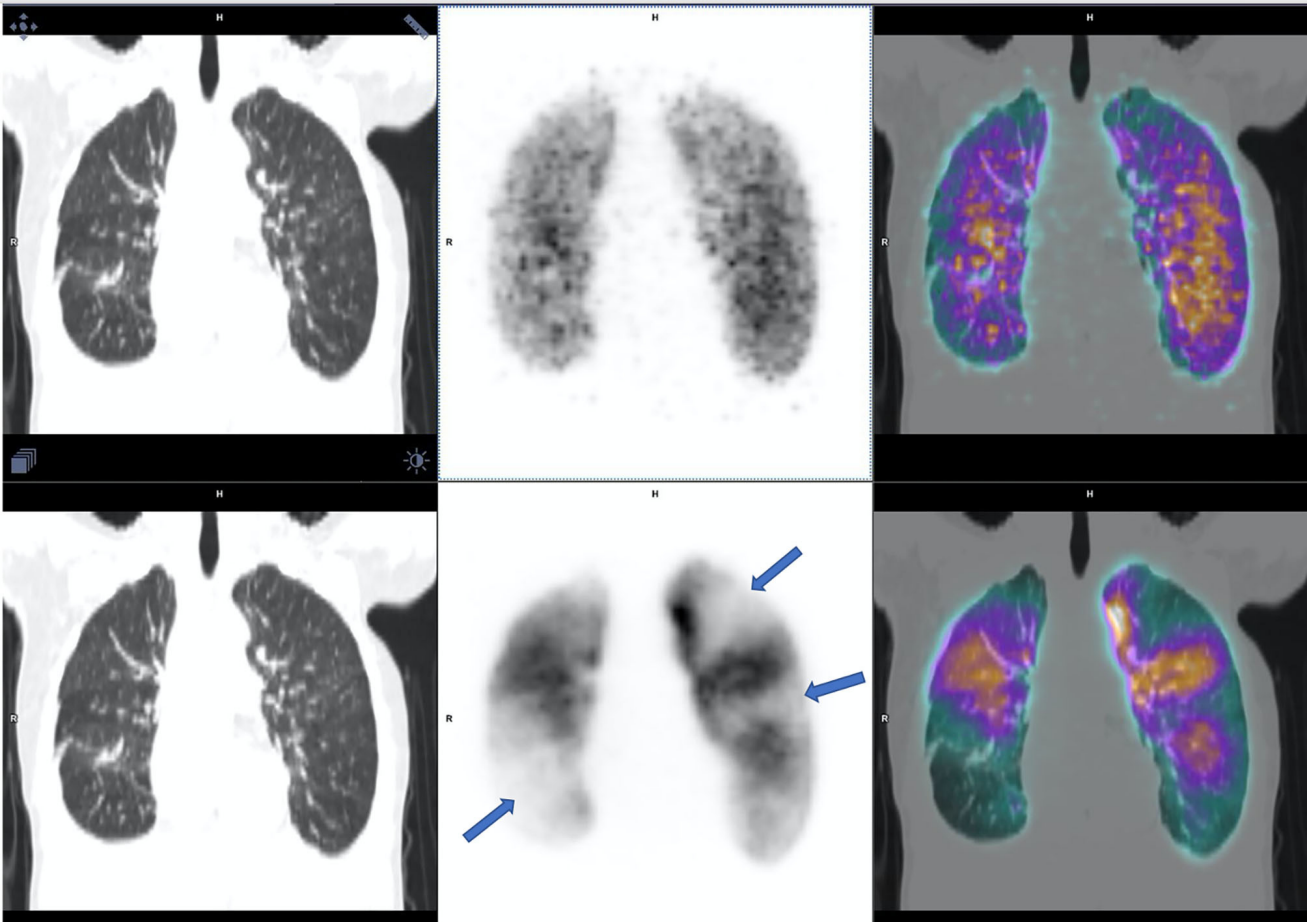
V/Q PET-CT, ventilation on the top and perfusion on the bottom, interpreted as positive by all of the four nuclear physicians with multiples mismatched perfusions defects (arrows).
